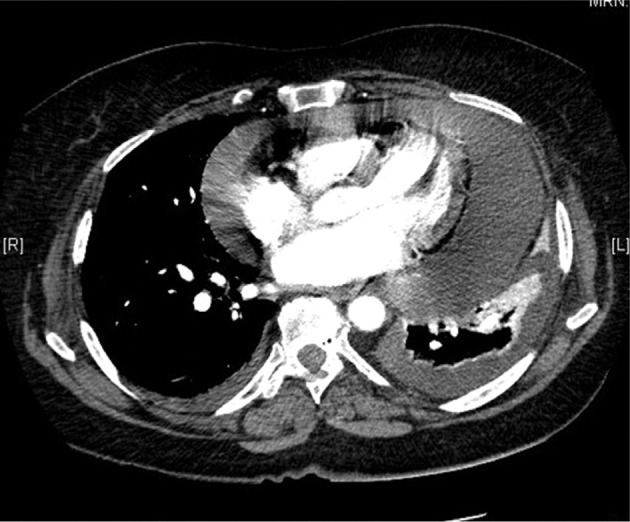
Computed tomography scan revealing a large circumferential pericardial effusion with bilateral pleural effusions.
